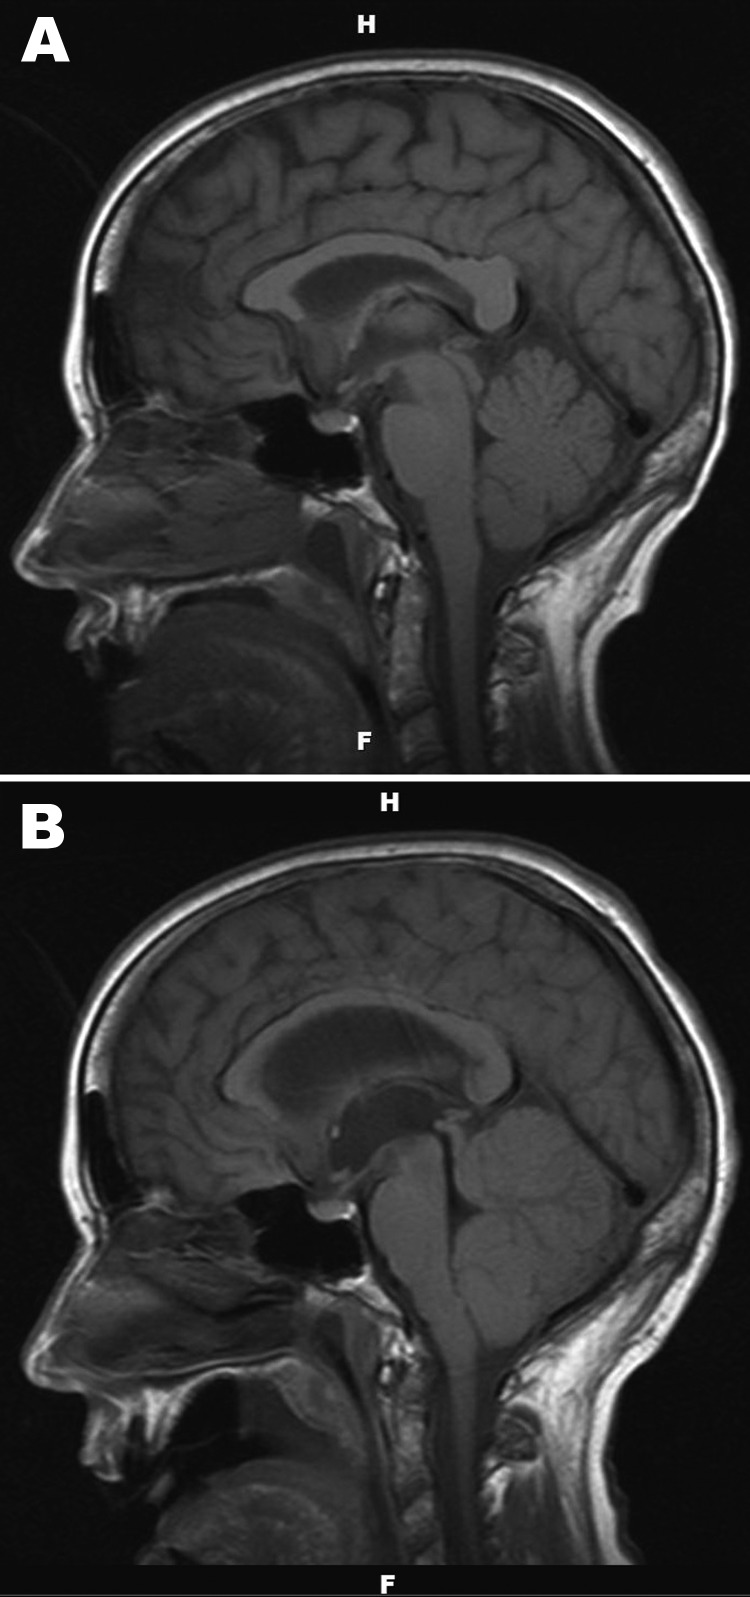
A) Noncontrast, sagittal T1-weighted magnetic resonance image of the brain of a 67-year-old woman with suspected Powassan virus encephalitis, obtained 4 days after admission. Image is notable for nonspecific signal changes within the thalami, midbrain, cerebellar vermis, and both cerebellar hemispheres. B) Noncontrast, sagittal T1-weighted magnetic resonance image of the brain obtained 8 days after patient’s admission. Changes include marked interval progression of signal abnormality involving the cerebellum, thalamus, and midbrain. Mass effect within the posterior fossa and crowding of structures at the foramen magnum are also evident. Marked dilatation of the lateral and third ventricles with acute hydrocephalus is apparent.
